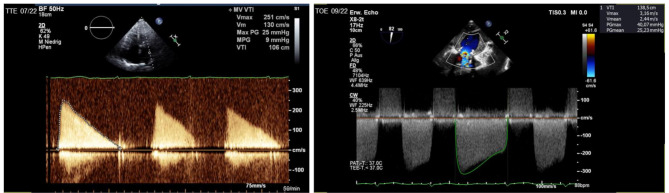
Mitral valve flow profile in CW doppler before (TTE) and after stenosis (TOE). In July.2022, there was a mean Gradient of 9 mmHg, in September 2022, there was a mean Gradient of 25 mmHg
